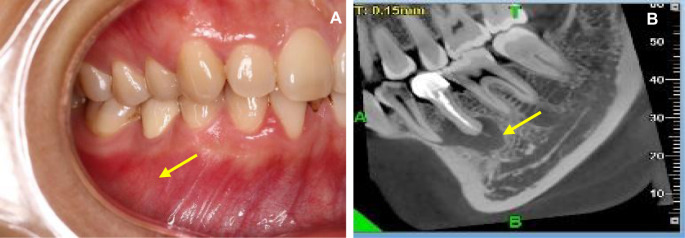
Buccal image and CBCT (NewTom 5G Version FP) examination. (A) Buccal image of the affected tooth. (B) CBCT image of the lesion area.
